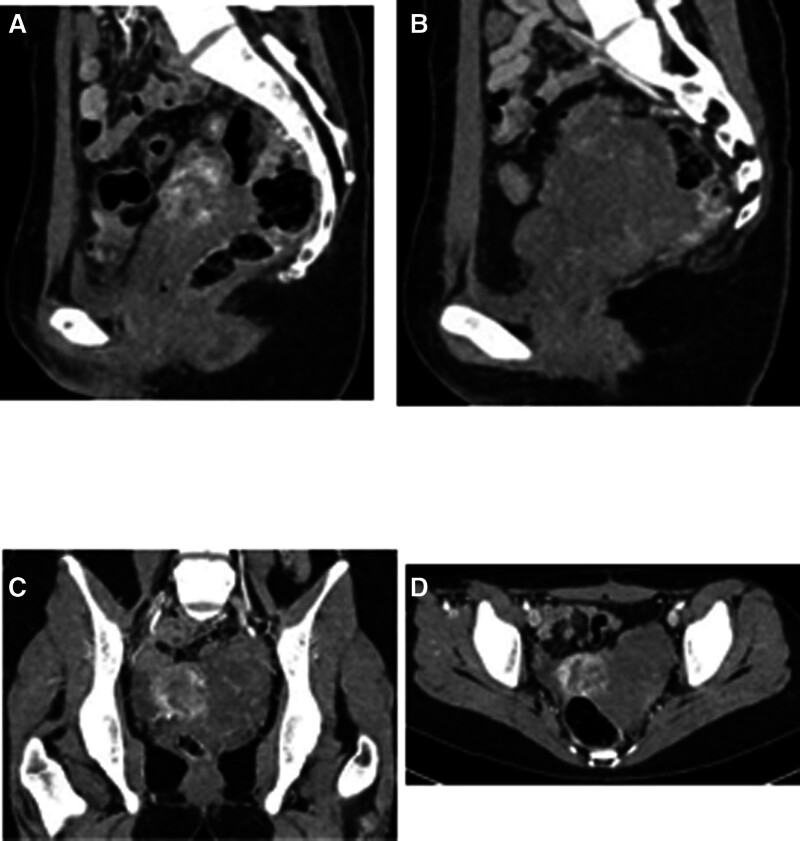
Enhanced CT images: (A) sagittal view showing bilateral ovaries and pelvic occupancy; (B) sagittal view displaying the maximum diameter of the pelvic occupancy in this section; (C) coronal view in the arterial phase; (D) axial view. CT = computed tomography.
